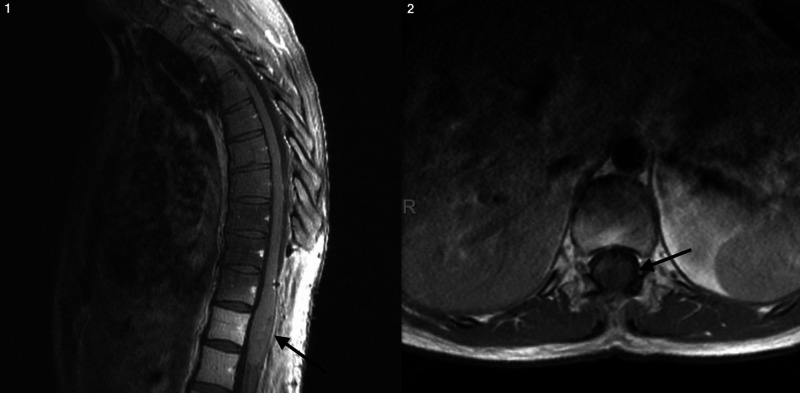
Sagittal (1) and axial (2) T1 MRI with contrast demonstrating an intramedullary tumor (arrow) that was found to be a WHO Grade II astrocytoma Abbreviations: S, superior; I, inferior; L, left; R, right; A, anterior; WHO, World Health Organization
